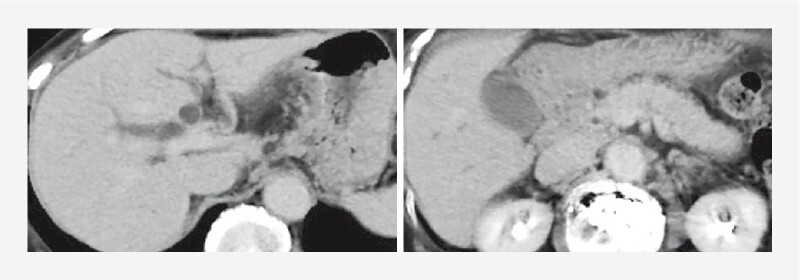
Abdominal contrast-enhanced computed tomography showed diffuse enlargement of the pancreas, distal bile duct stenosis, and intrahepatic bile duct dilation.
